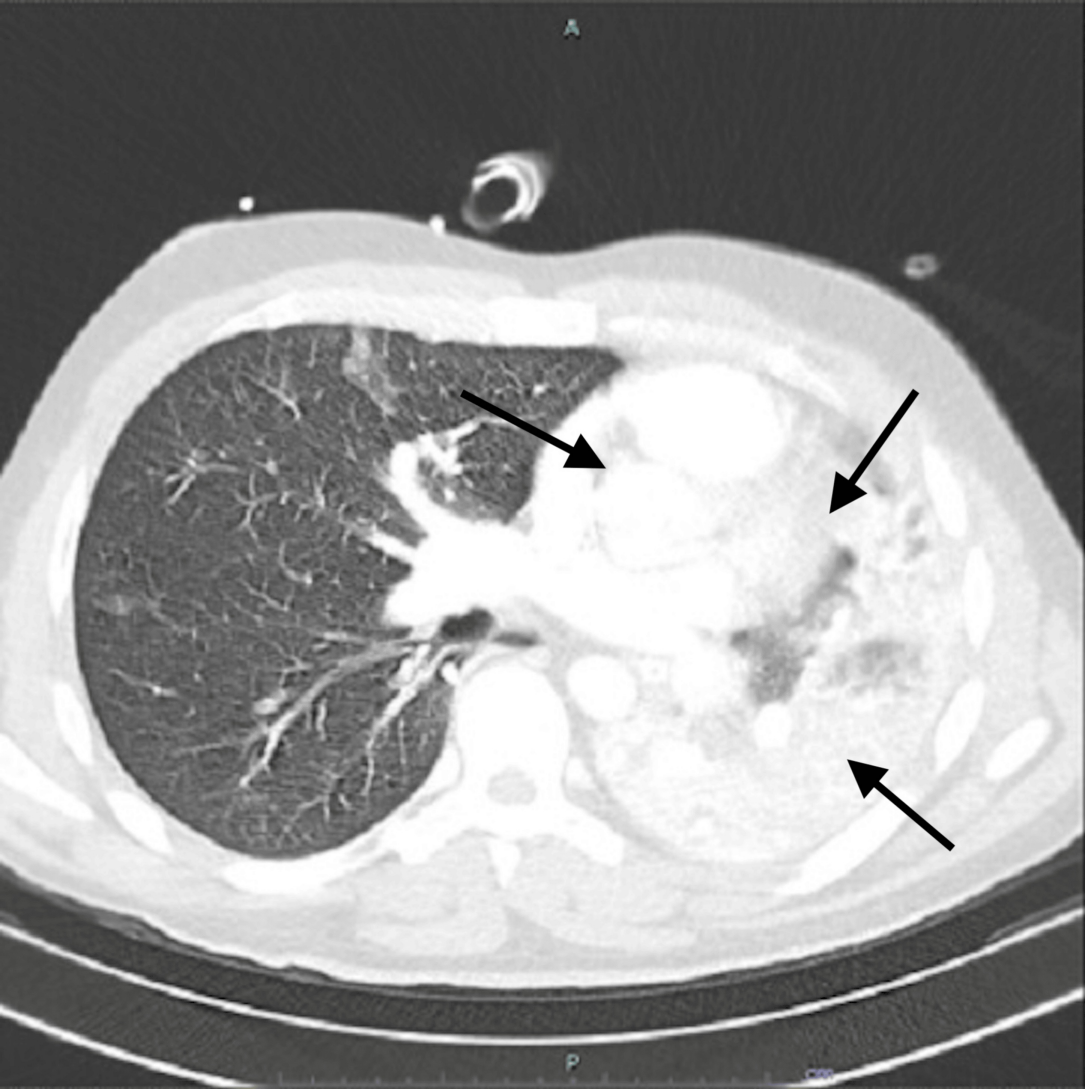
Chest CT (axial view) showing reduced volume of the left lung with near-total involvement by confluent consolidations extending to the pulmonary periphery, consistent with diffuse alveolar hemorrhage.
